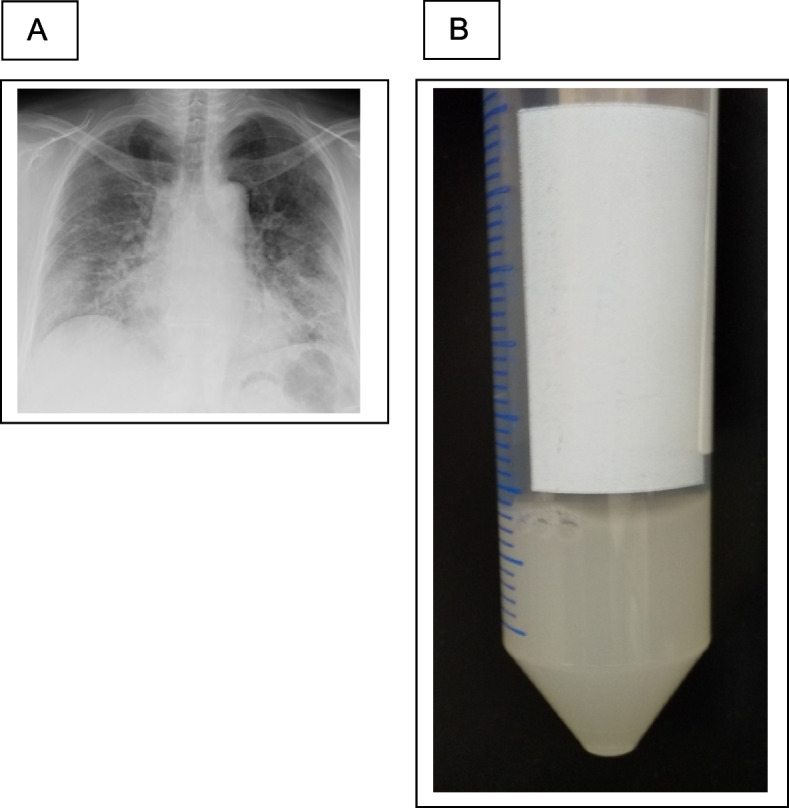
Chest x-ray on admission for bronchoscopy and bronchoalveolar lavage fluid (BALF). A Chest radiograph before bronchoscopy showing ground-glass shadows in both lungs. B The collected alveolar lavage fluid was thick and milky white in color
